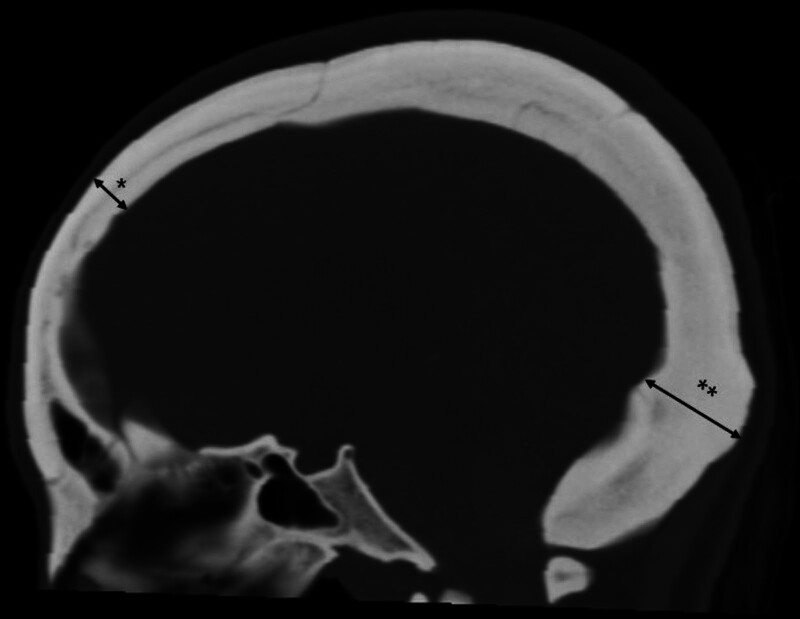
Computed tomography prior to the first-stage decompressive surgery, demonstrating diffuse cranial bone thickening with the characteristic “marble bone” appearance, a diagnostic hallmark of osteopetrosis. Calvarial thickness measures approximately 7 to 8 mm in the frontal region (*) and up to 29 mm in the suboccipital area (), contributing to significant posterior fossa crowding.
